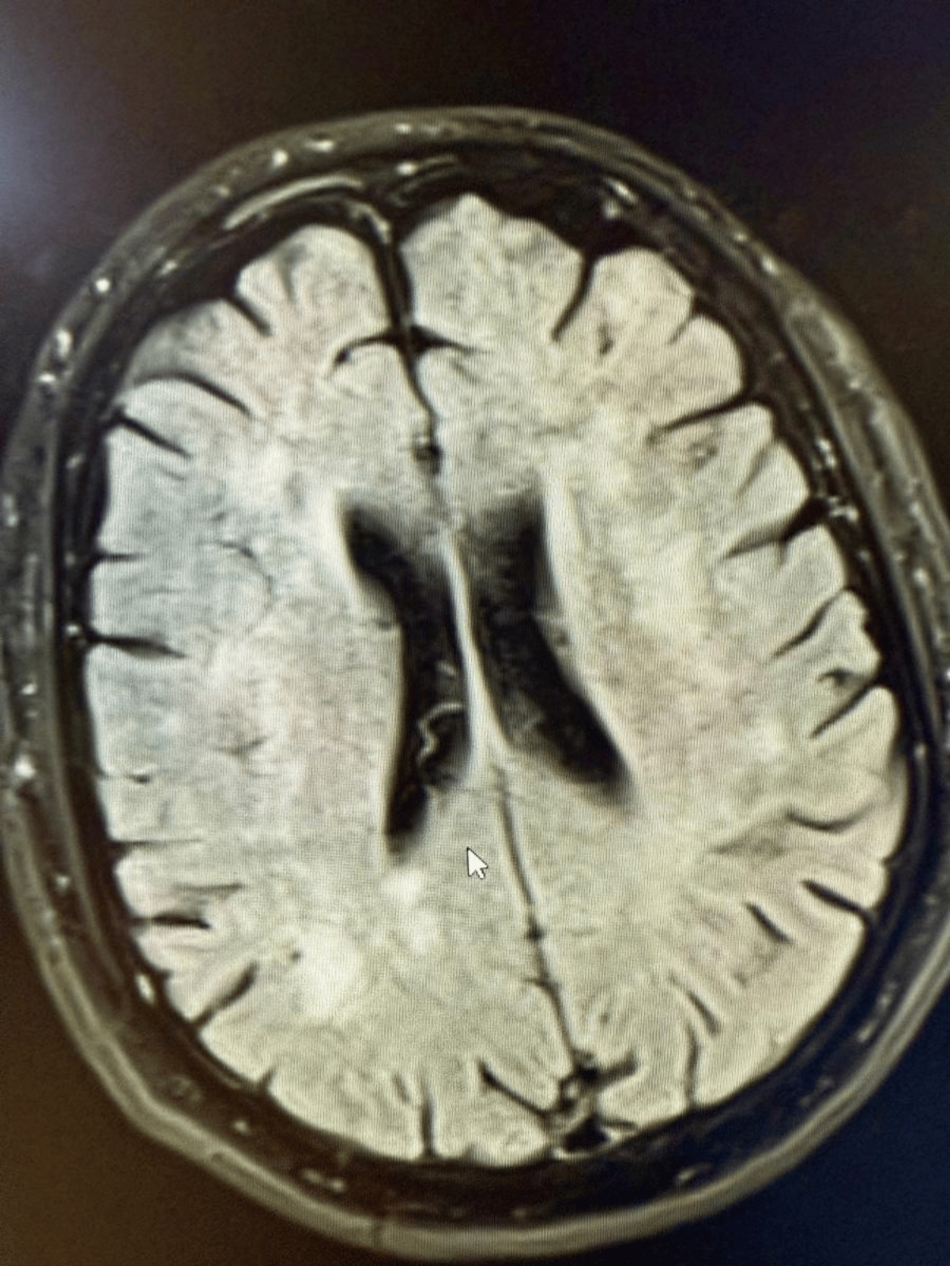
MRI of the brain showing acute infarctions
